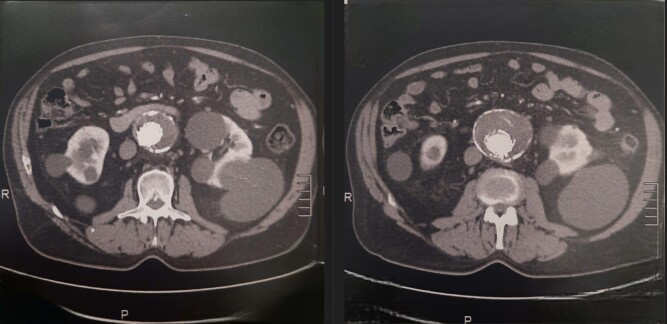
Computed tomography with contrast showing absence of blood flow into the aneurysm sac, in 2023.
